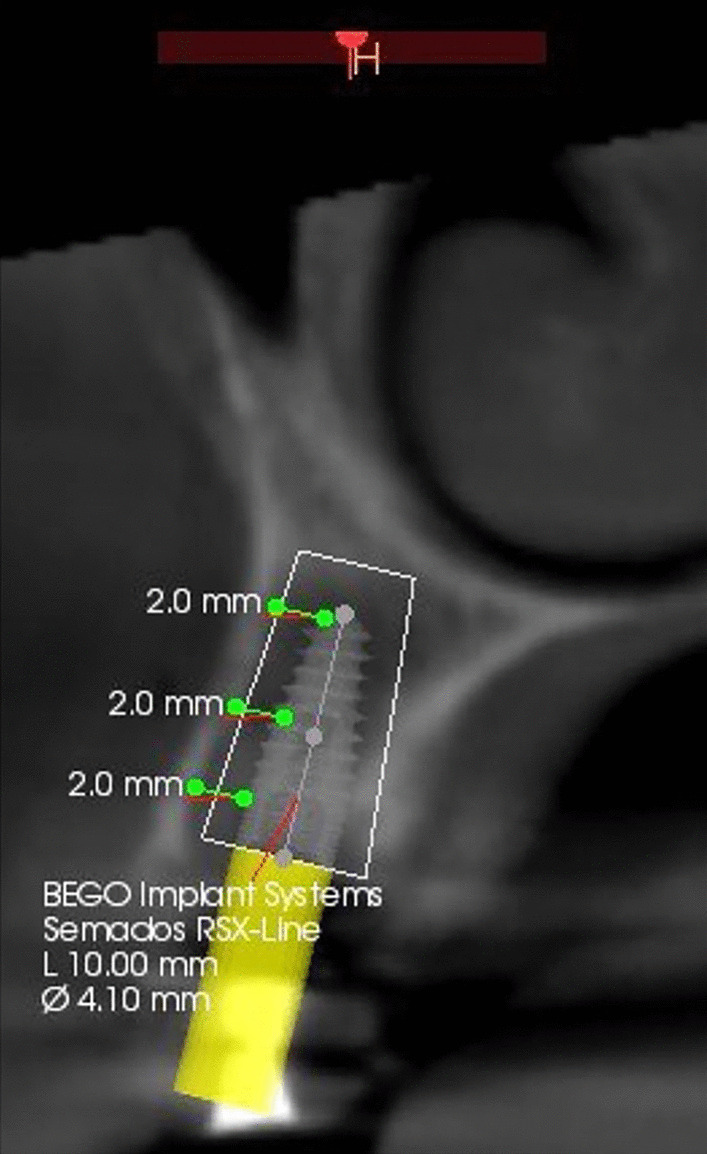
CBCT Para-axial view showing preoperative bone density measurements
